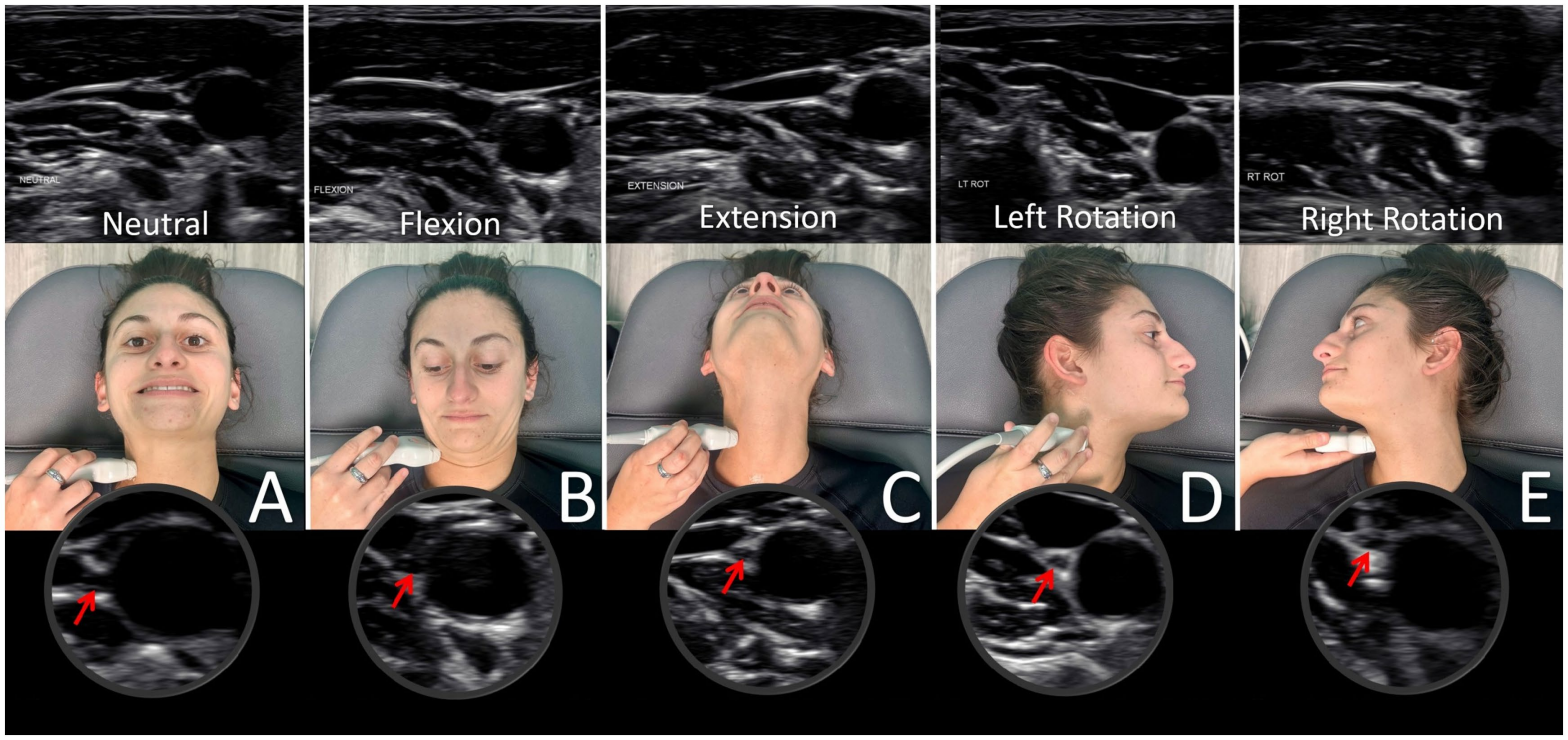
Ultrasound of vagus nerve in mid-cervical region with various neck positions. (A) Neck in neutral position. (B) Neck flexed. (C) Neck extended. (D) Neck rotated left. (E) Neck rotated right. As can be seen, the vagus nerve within the carotid sheath undergoes various structural tensions depending on neck positions.
